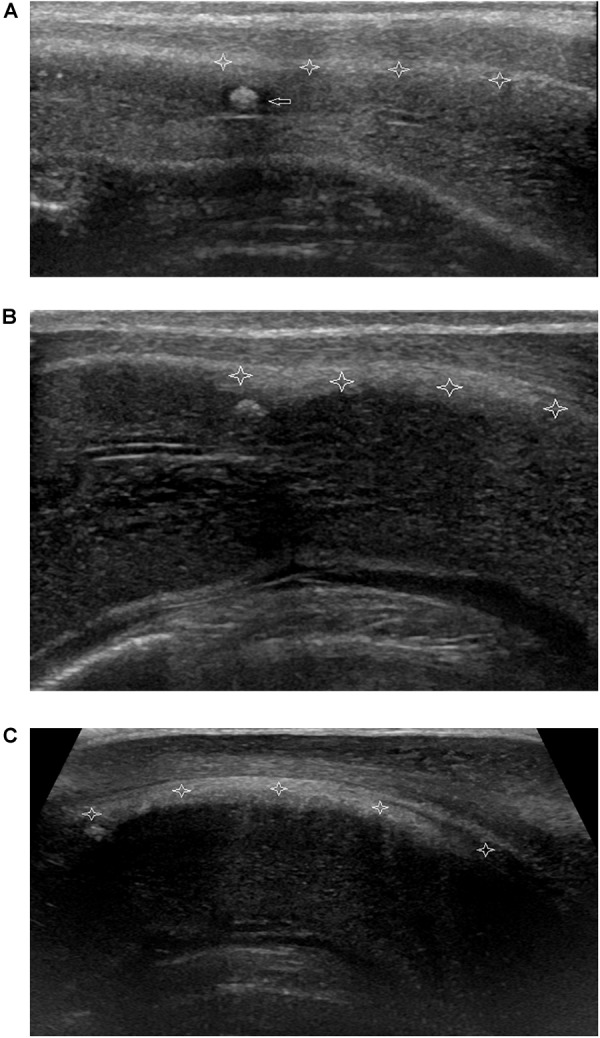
Non-palpable nodule of focal thickening of the ventral tunica albuginea of corpora cavernosum behind corpora spongiosum at the root of penis. (A) Longitudinal sonogram, ventral access. No focal thickening of tunica albuginea of corpora cavernosum (stars) is shown and a hyperechoic fibrous plaque (arrow) in the corpora cavernosum in flaccid penis. (B) Longitudinal sonogram, ventral access. With the corpora cavernosa expansion, the local tunica albuginea shows thickening (stars) with the weak acoustic attenuation in the erect penis. (C) Longitudinal sonogram, ventral access. The acoustic attenuation behind the thickening tunica albuginea becomes strong when the penis reaches complete erection.
